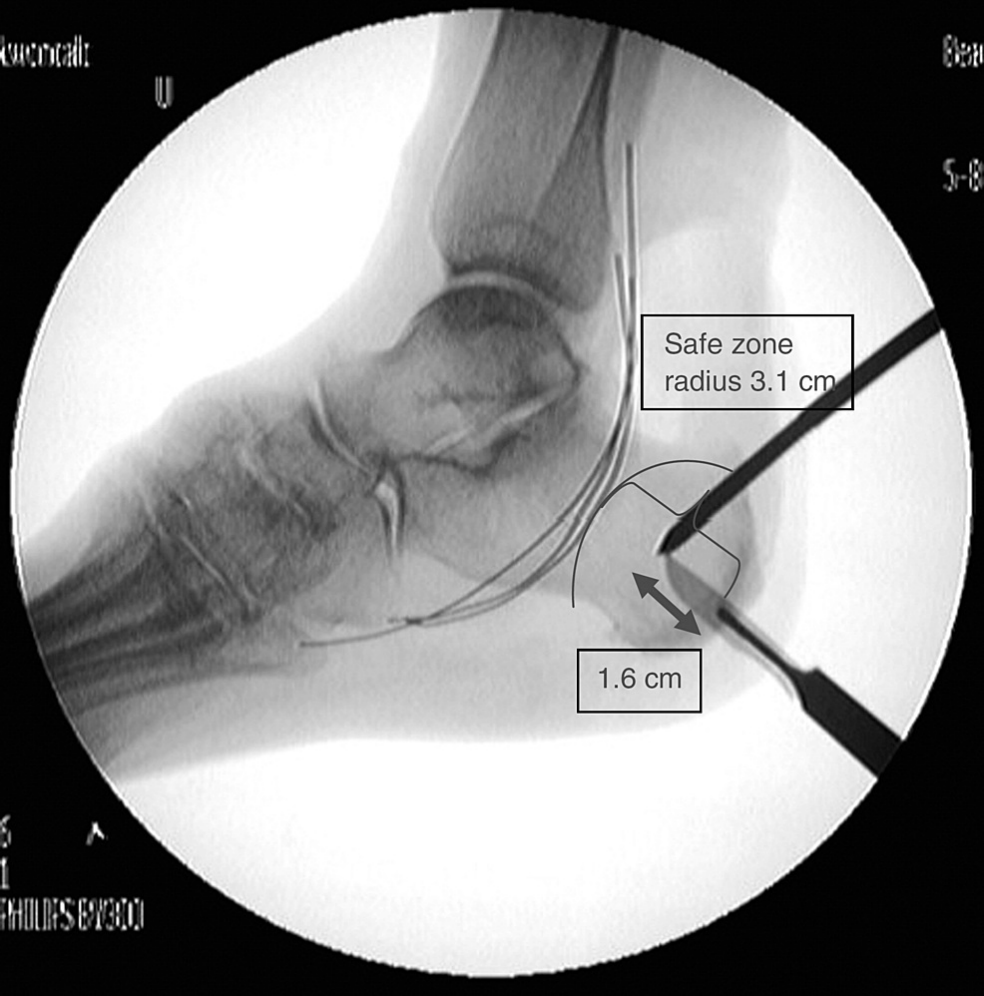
Radiographic image of the anatomic safe zone.
